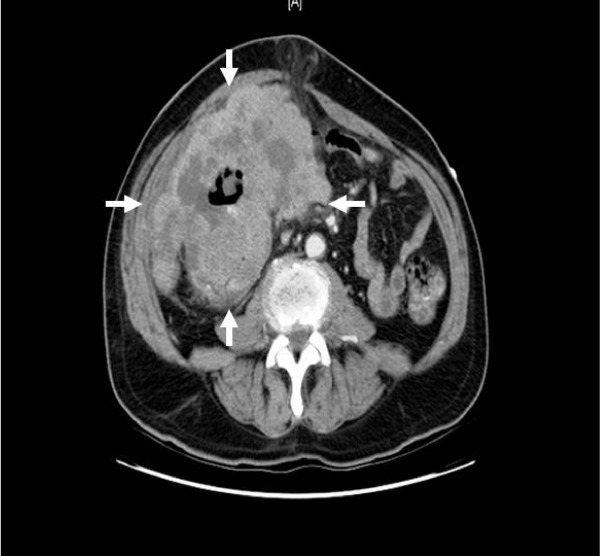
Axial view of Computed Tomography (CT), which revealed 13 × 12 × 16 cm right-sided ill-defined soft tissue mass involving the ascending colon.
